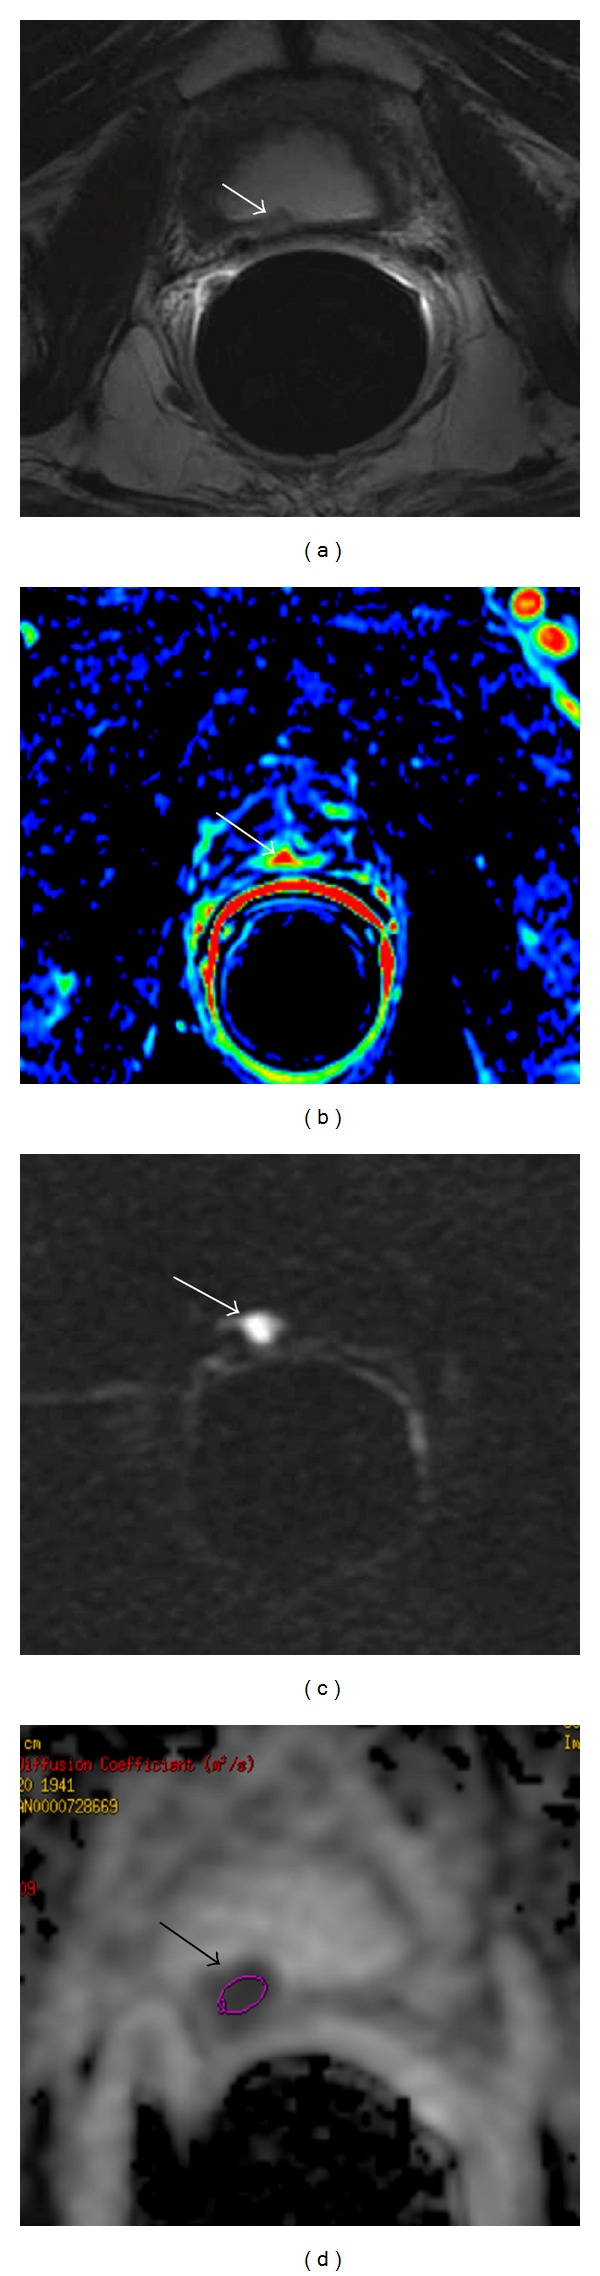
Multiparametric-MR images of a 74-year-old man with prostate-specific antigen progression (PSA serum level 0.43 ng/mL) after radical retropubic prostatectomy, with suspected local recurrence. (a) Axial T2-weighted fast spin-echo image shows a solid nodular tissue of about 7 mm in size on the right posterior perianastomotic location in front of the rectal wall at about 12 mm from the ureteral meatus which is slightly hyperintense compared to pelvic muscles (white arrow). (b) Axial gradient-echo T1-weighted color map image showing a remarkable enhancement of the pathological tissue (white arrow). (c) Axial native DWI image at *b* value = 3,000 s/mm^2^ showing marked restricted diffusion of water molecules (white arrow). (d) Axial ADC map reconstructed from images obtained at *b* values of 0, 500, 1,000, and 3,000 s/mm^2^ shows a dark area corresponding to the abnormal hyperintense tissue seen on T2-weighted images and hypervascular nodule seen on color map (black arrow). All these findings are consistent with locoregional relapse.
